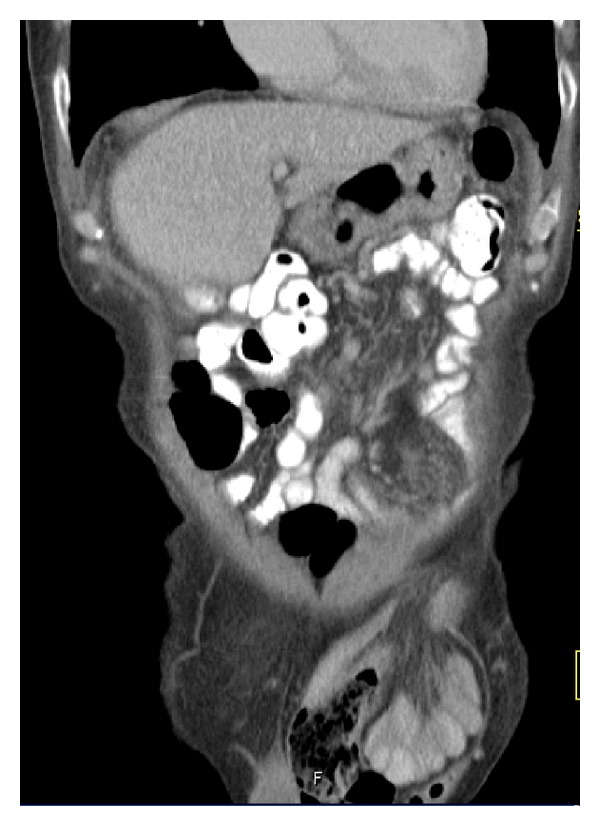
Coronal CT showing herniation of colon and mesentery through the inguinal canal.
